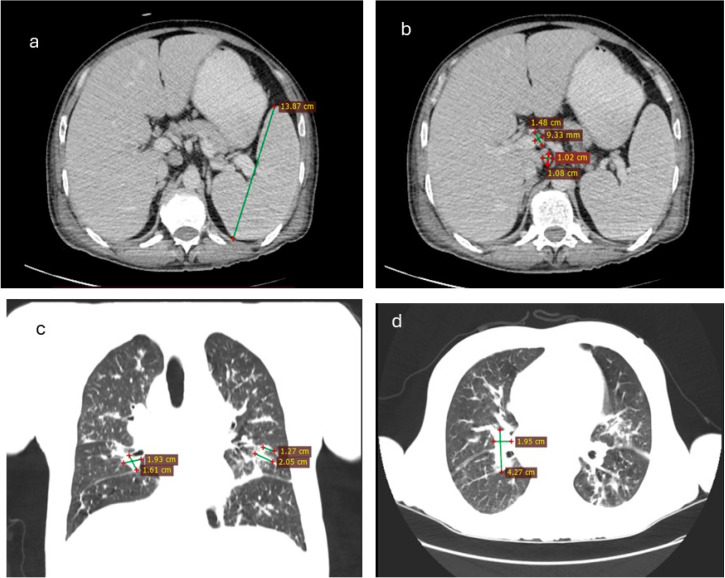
CT scanner particularities: (a) splenomegaly; (b) adenopathies in the liver hill; (c) fibrosis bands in the lungs (axial); (d) fibrosis bands in the lungs (sagital).
